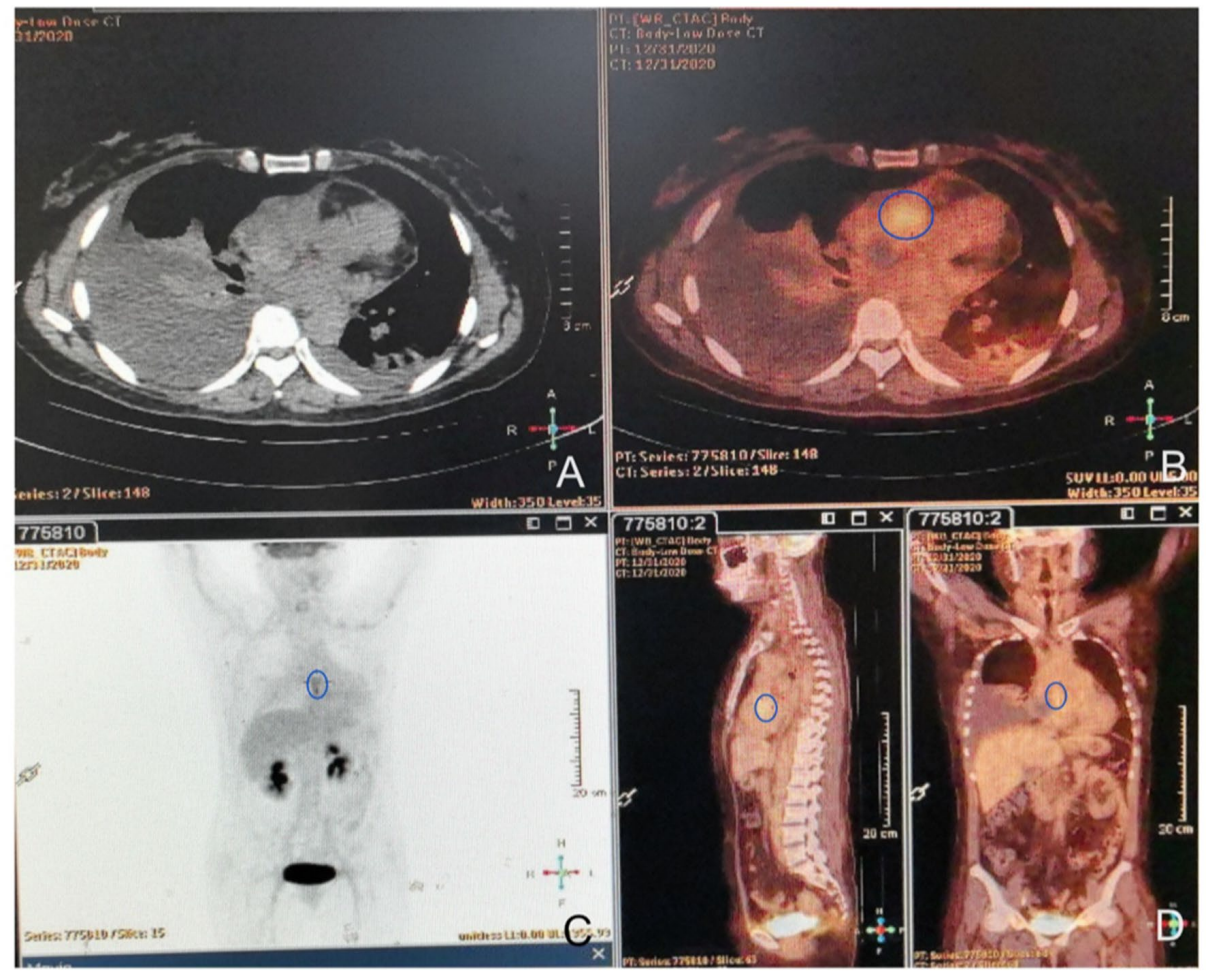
FDG-PET/CT images. (A) On the unenhanced CT, the mass was seen to invade the mediastinum and was contiguous with the pericardium. (B) Axial FDG/CT image. Mild uptake in the mass with an SUV_max_ of 4.2 SUV (circle area) was detected, and no extra-cardiac lesions were observed. (C,D) Whole-body maximum intensity projection (MIP) images show heterogeneous uptake (circle areas) in the expected location of the right heart.
